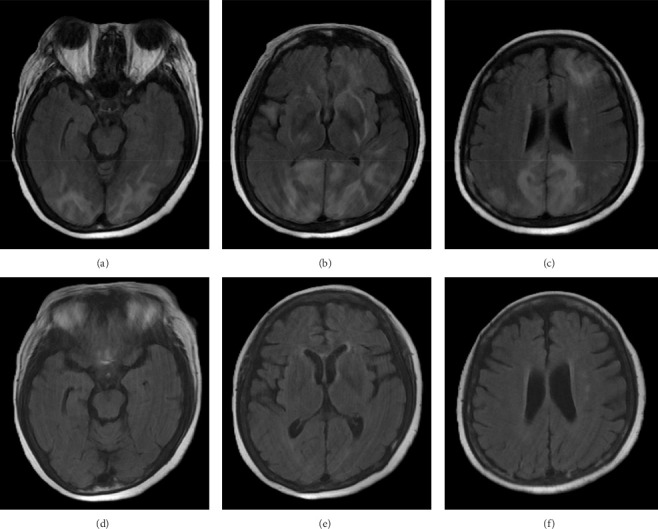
Brain MRI (FLAIR sequences) before and after treatment. (a–c) Axial FLAIR images obtained during the acute episode show hyperintense signals in the bilateral occipital, parietal, and left frontal lobes. Images are arranged from inferior (a) to superior (c). (d–f) Follow-up images acquired 4 months after treatment demonstrate complete resolution of the previously noted abnormalities. Images are arranged from inferior (d) to superior (f). Abbreviations: FLAIR: fluid-attenuated inversion recovery; MRI: magnetic resonance imaging.
